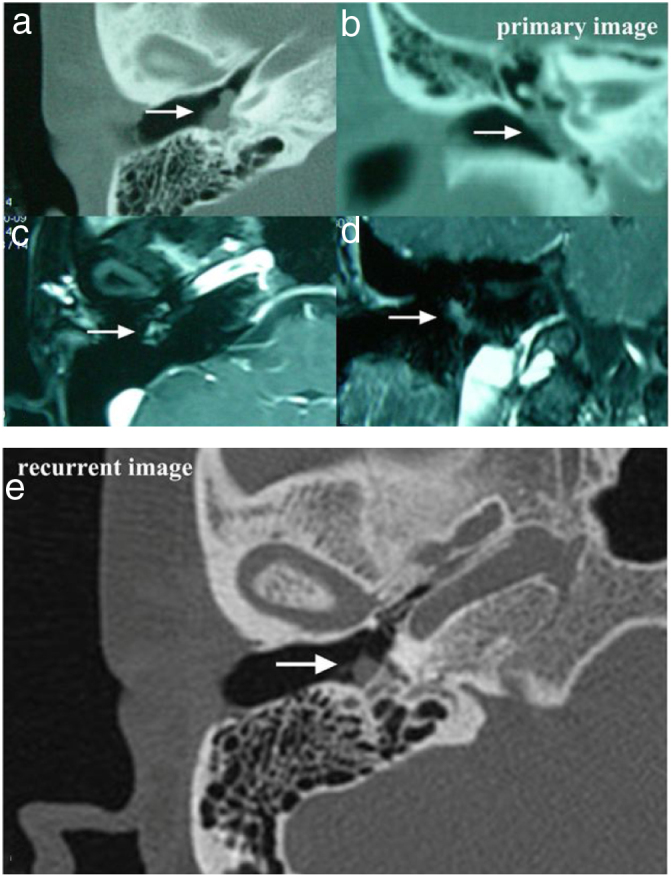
The temporal bone CT and MRI of Case 3. Images A, B, C, D showed the lesion was located in the meso- and hypotympanum with ossicular chain encasement on temporal bone CT (A, axial; D, coronal), and moderately enhance with gadolinium administration on MRI (C, axial; D, cronal). Image e showed tumor recurrence on high resolution temporal bone CT (E, axial), it demonstrated an isointensity mass located in the tympanum without bone erosion.
